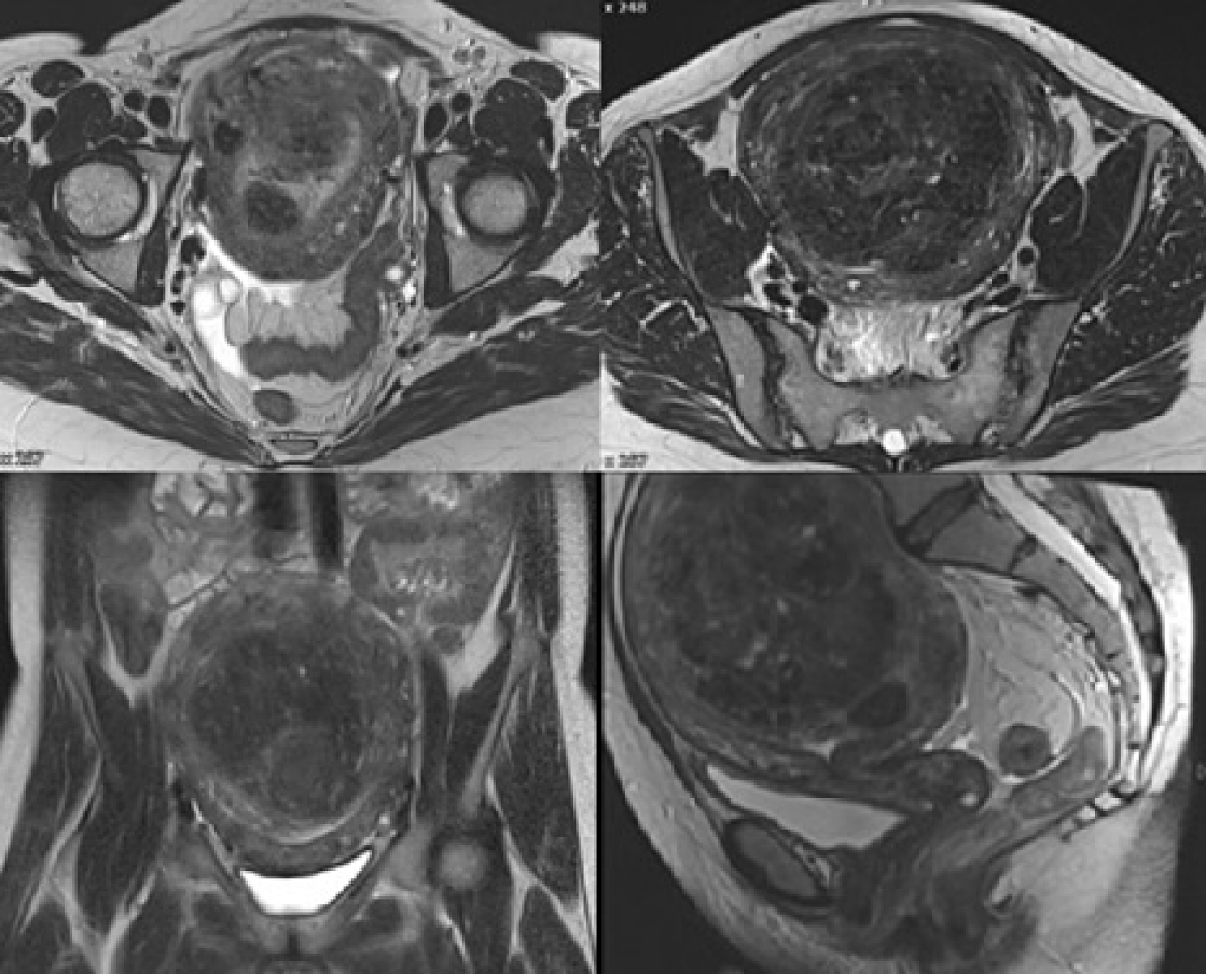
MRI with large myomatous uterus
